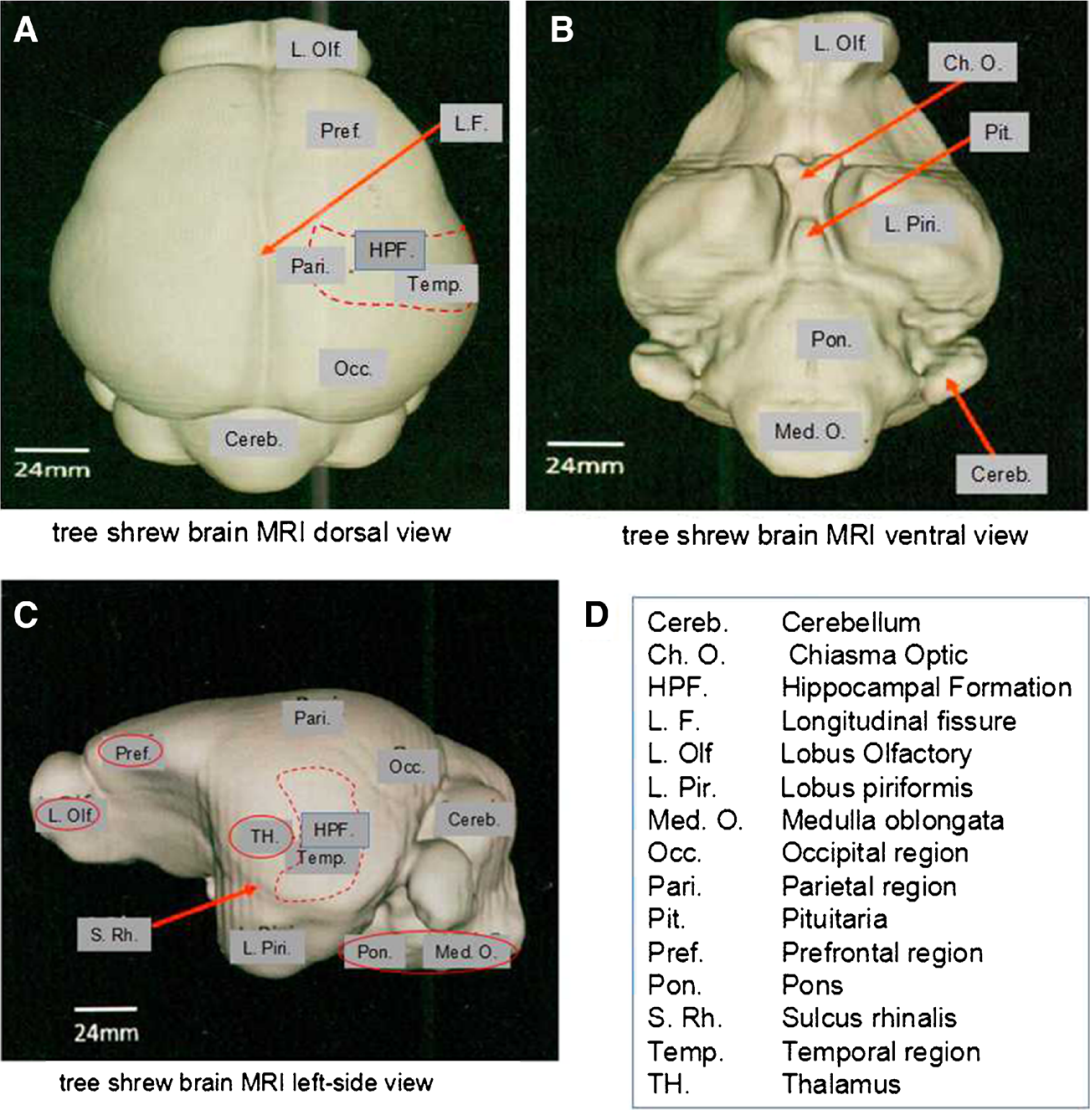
3-D view of the tree shrew brain. (adapted from Basic Biology and Disease Models of Tree Shrews (Zheng et al. 2014). Copy right release obtained from Kunming Institute of Zoology, Chinese Academy of Sciences). a Tree shrew brain MRI 3-D reconstruction dorsal view. b Tree shrew brain MRI 3-D reconstruction ventral view. c Tree shrew brain MRI 3-D reconstruction left-side view. d Different regions of the brain are marked. Dissected regions of the brain for experiments described in this study are *circled in red. Dashed circled areas* (HPF) marked areas that are beneath the surface of the brain
